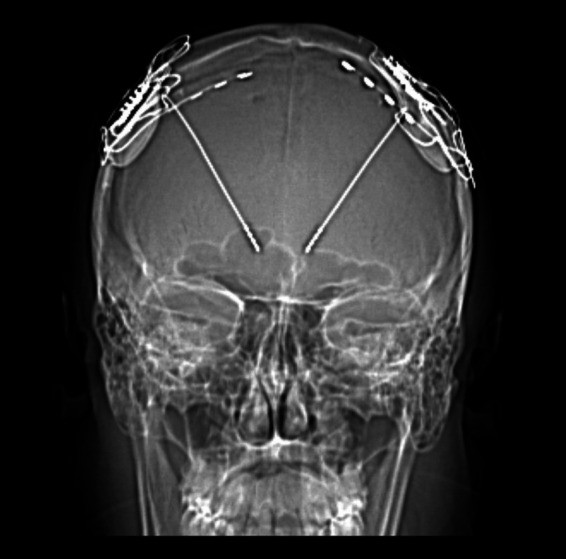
Frontal X-ray depicting DBS electrodes implanted in the bilateral thalamus, and neocortical strip electrodes.
